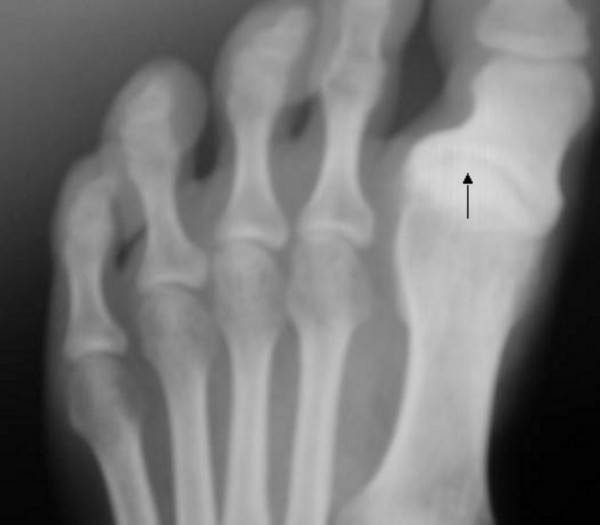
Patient 1: Anteroposterior at age of 17 years foot radiograph showed progressive hallux varus with marked osteoarthritis of the base of the first toe.
